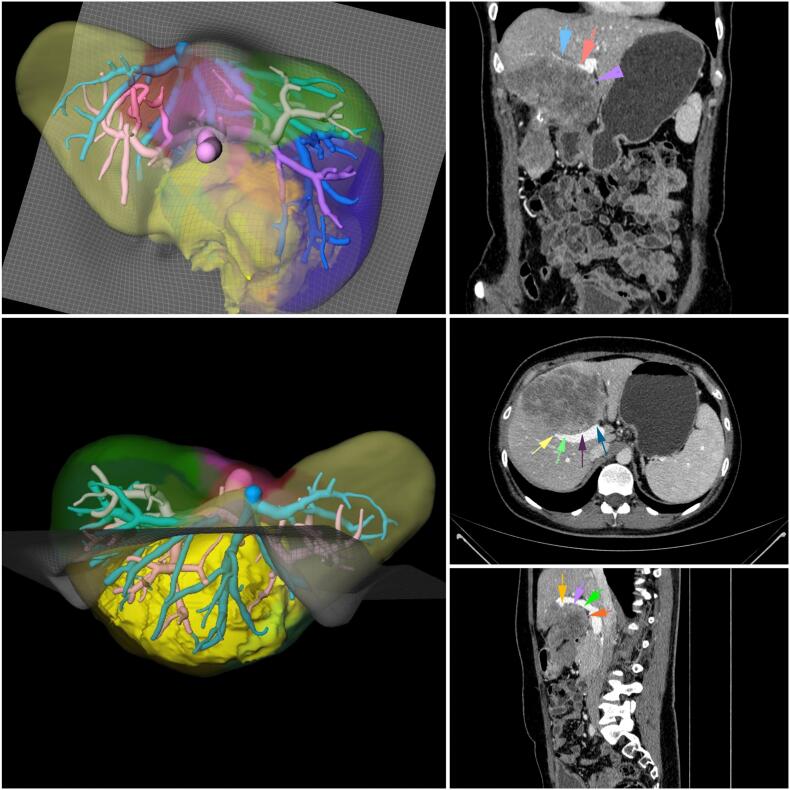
2D and 3D CT imaging of the patient after 3 months of CH.
